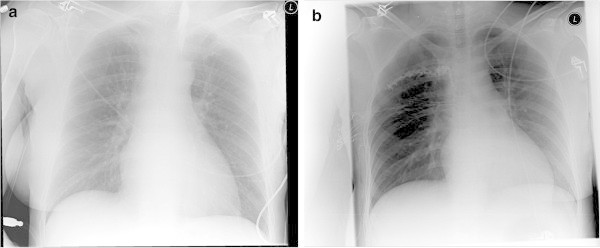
Examples radiographic contrast medium spread in patients with continuous paravertebral block. a. radiographic contrast medium spread in thoracic paravertebral space. b. Intercostal spread of radiographic contrast medium.
